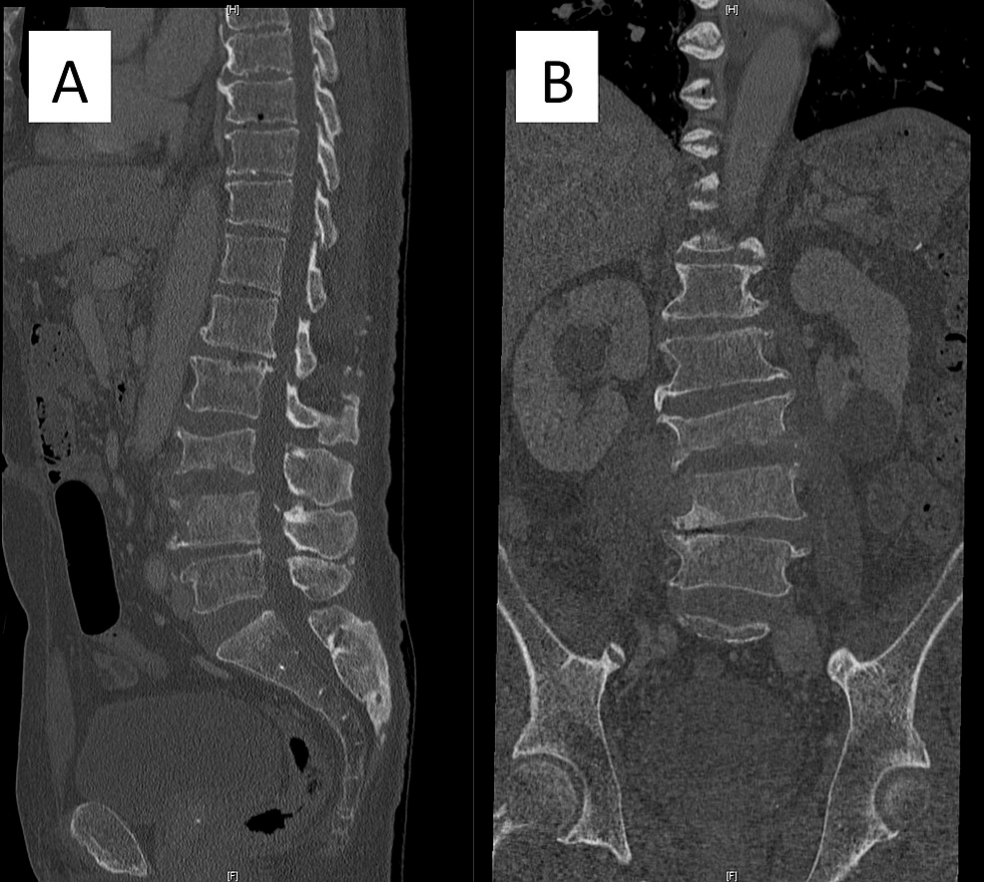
Secondary computed tomography scan slices after four weeks of antibiotics administration. (A) Sagittal slice. (B) Coronal slice. Progressive destructive changes of the L3 and L4 endplates and vertebral body were observed.
